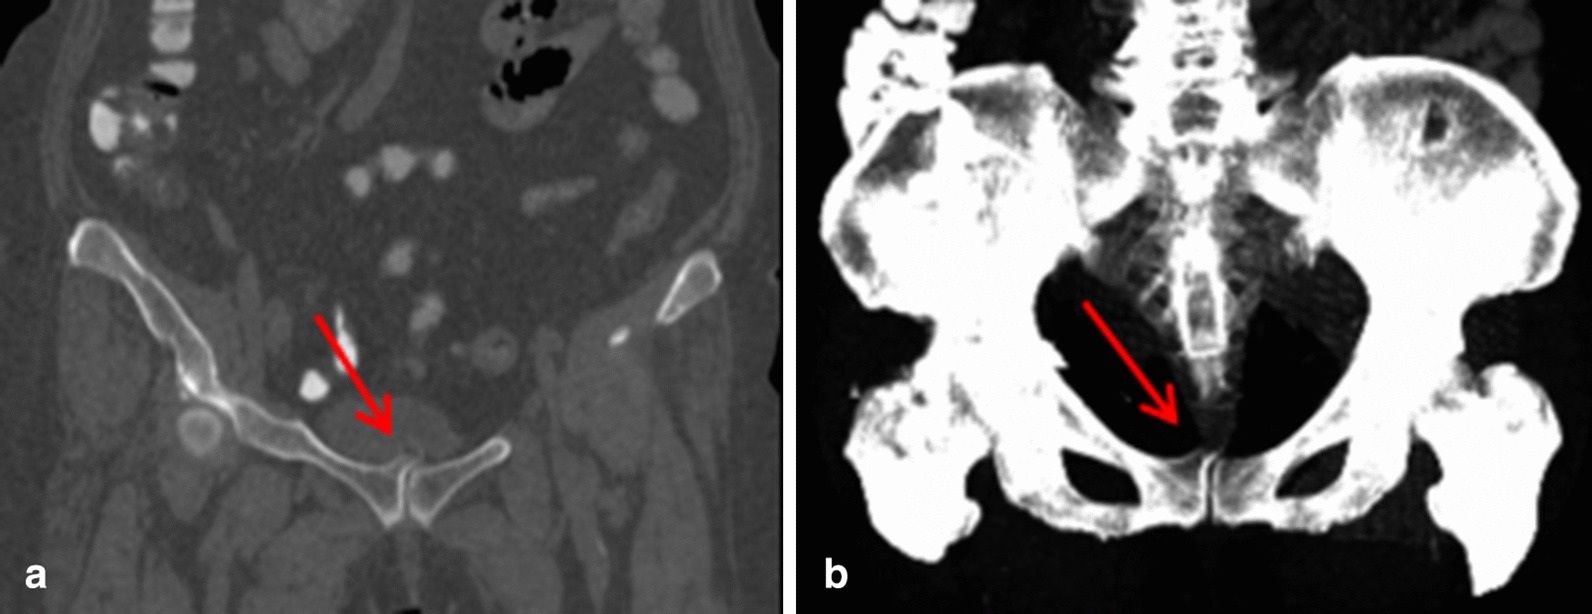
a Coronal CT showed that a lesion arose from the superior aspect of the right pubic symphysis and grew towards the upper left region, impinging on the bladder (arrow). b The 3D reconstruction of the pelvis showed that the marrow and cortex of the bony mass were continuous with those of the adjacent normal bone (arrow)
